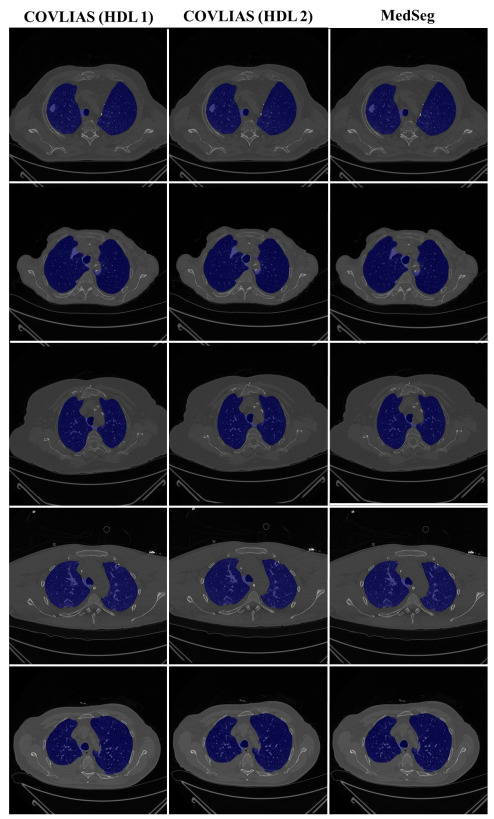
COVLIAS vs. MedSeg: Segmented mask (blue) on the non-COVID CT lung image (small lung).
